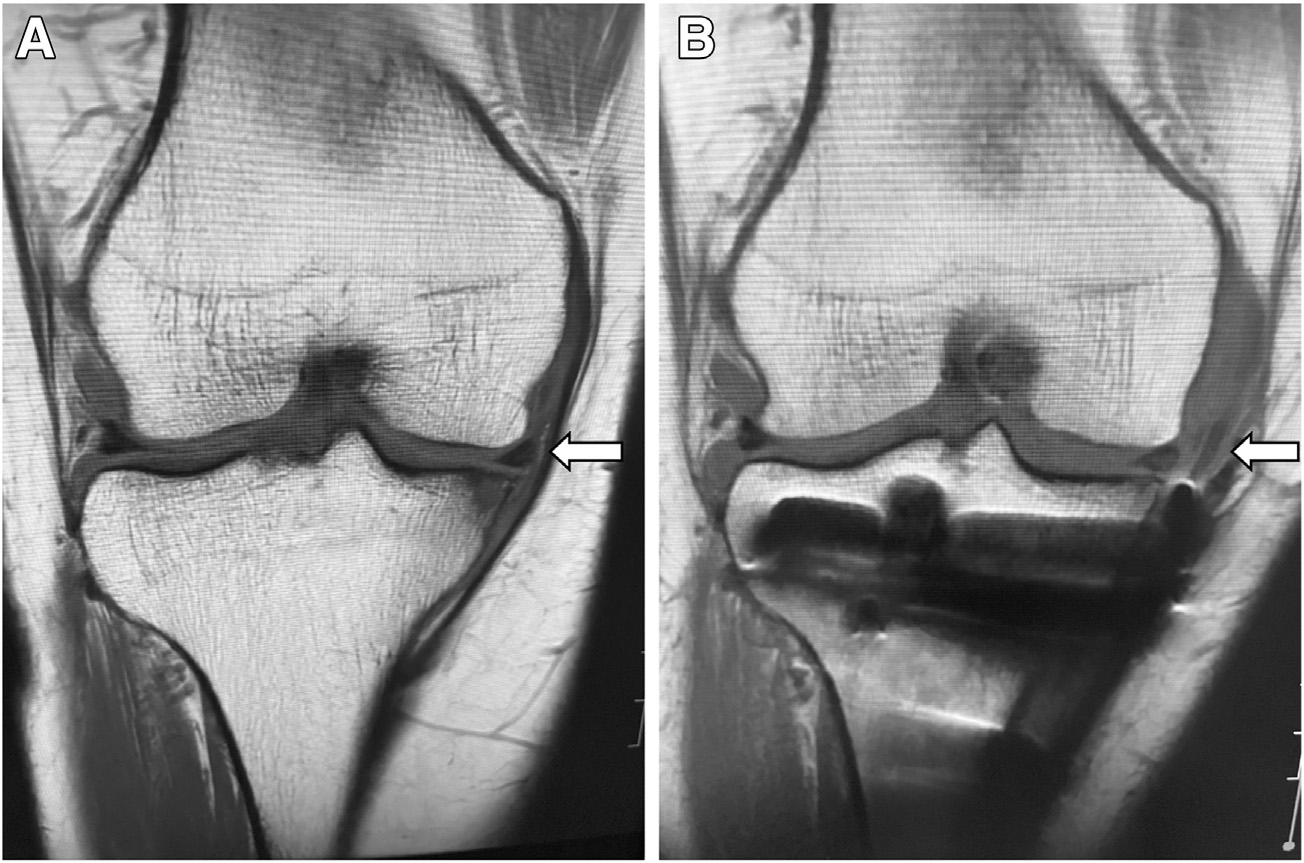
Preoperative (A) and postoperative (B) magnetic resonance images of right knee. Postoperatively, as indicated by the white arrows, the extrusion of the medial meniscus is corrected, and the graft created on the lateral side of the joint capsule is visible.
